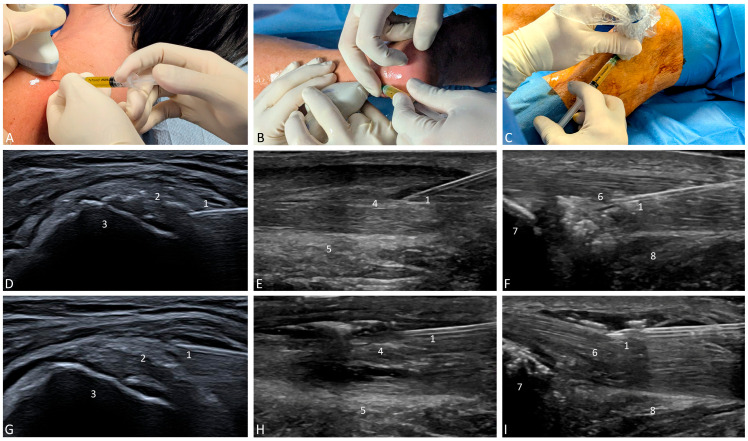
Application of PRP in tendinopathies. PRP was administered into the supraspinatus tendon of the rotator cuff (A,D,G), Achilles tendon (B,E,H), and patellar tendon (C,F,I). The injections were ultrasound-guided (A–C), using both an intratendinous (D–F) and peritendinous (G–I) approach. When administering PRP, a hyperechoic signal was observed. 1: needle; 2: supraspinatus tendon; 3: greater tuberosity; 4: Achilles tendon; 5: Kager’s fat pad; 6: patellar tendon; 7: patella; 8: Hoffa’s fat pad.
